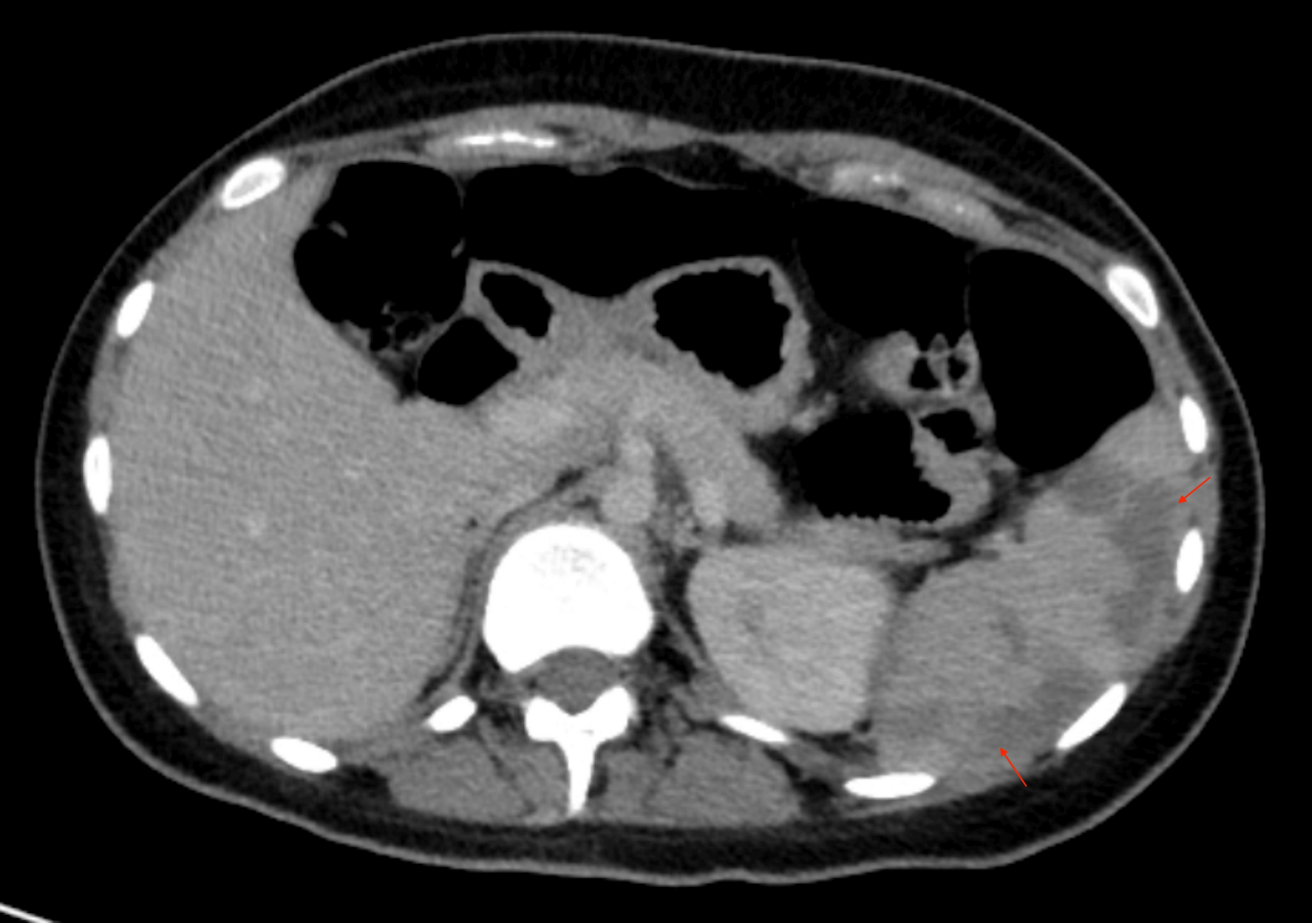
Abdominal CT with extensive splenic infarctions Contrast-enhanced axial CT image of the abdomen demonstrating multiple, wedge-shaped, hypodense areas (arrows) in the spleen.
